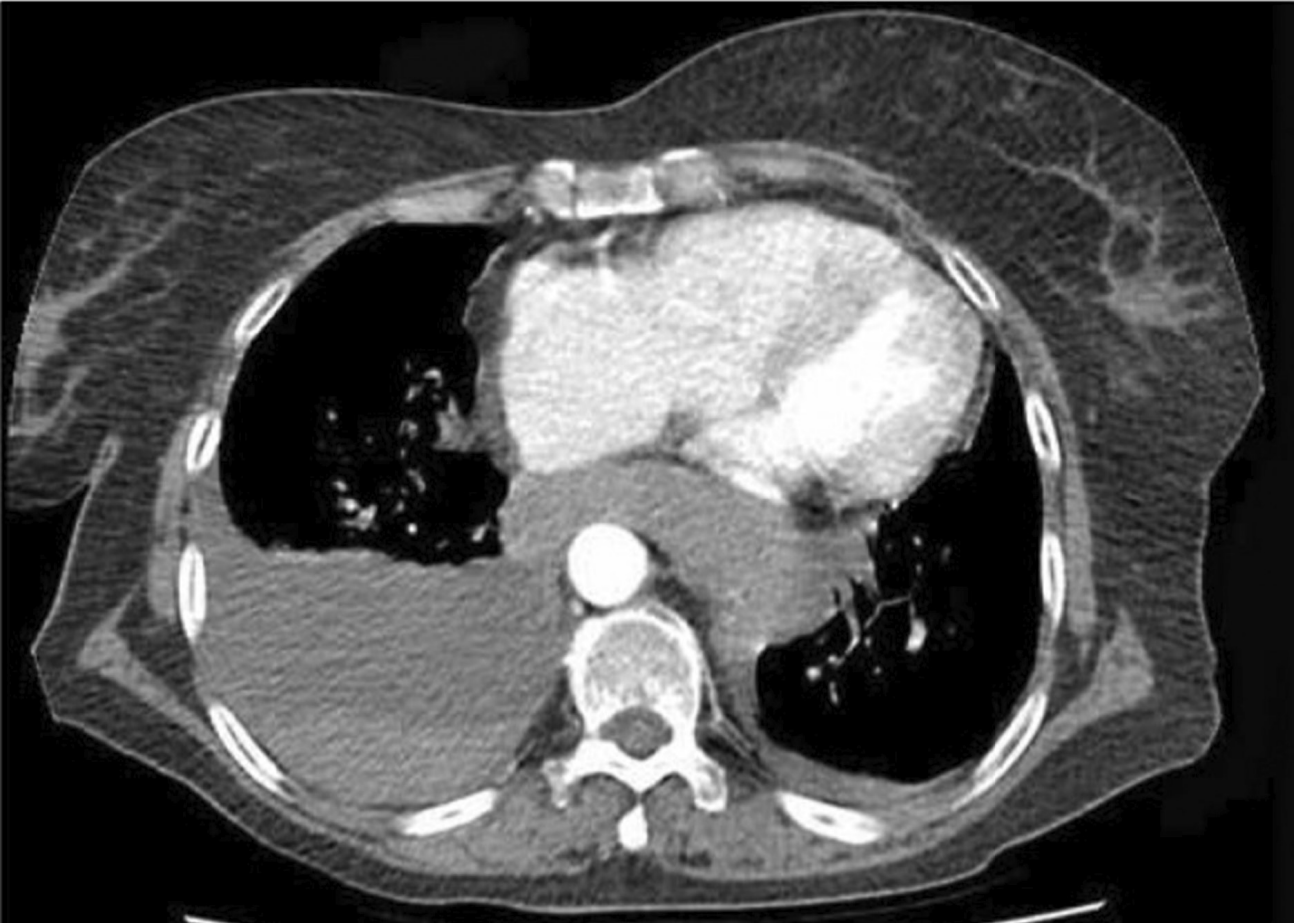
Chest CT reveals right‐side pleural effusion and mediastinal hematoma.
